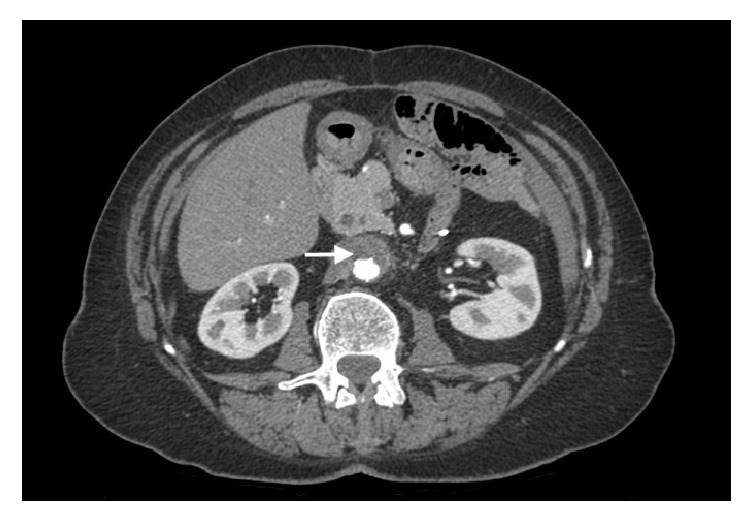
Abdominal CT scan (day 6) showing an increased periaortic infiltration highly suggestive of infectious aortitis (arrow).
